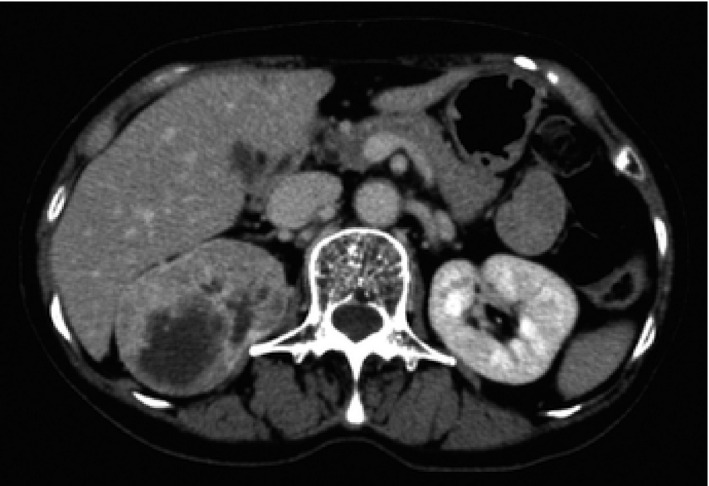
CT shows a large mass with early enhancement in the right kidney with tumor thrombus in the right renal vein.
